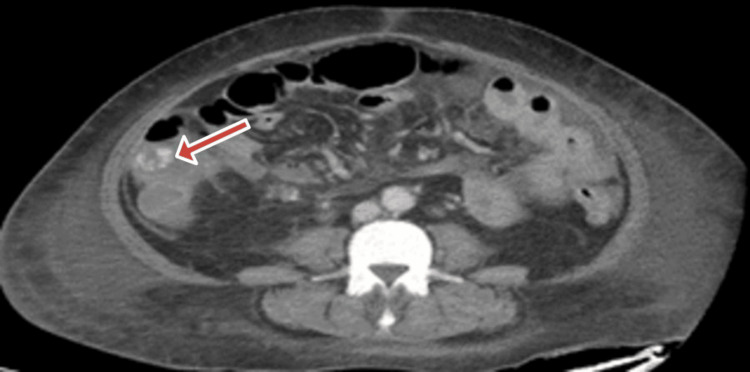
CT abdomen and pelvis, showing active contrast extravasation in ascending colon (the white spot on the right upper corner)
